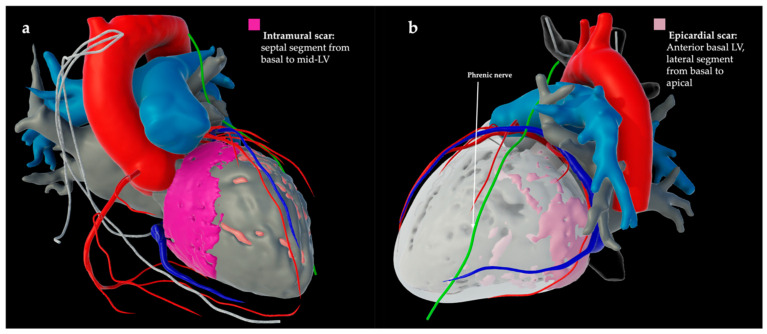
Late iodine enhancement CT (LIE-CT)-derived cardiac model after segmentation (inHEART, Pessac, France) of two patients with non-ischemic dilated cardiomyopathy. (a) Late iodine CT shows intramural scar (pink) in the septal left ventricle (LV) from basal to mid-LV. (b) Late iodine CT shows epicardial scar (light pink) on the anterior basal LV and the lateral segment from basal to apical.
